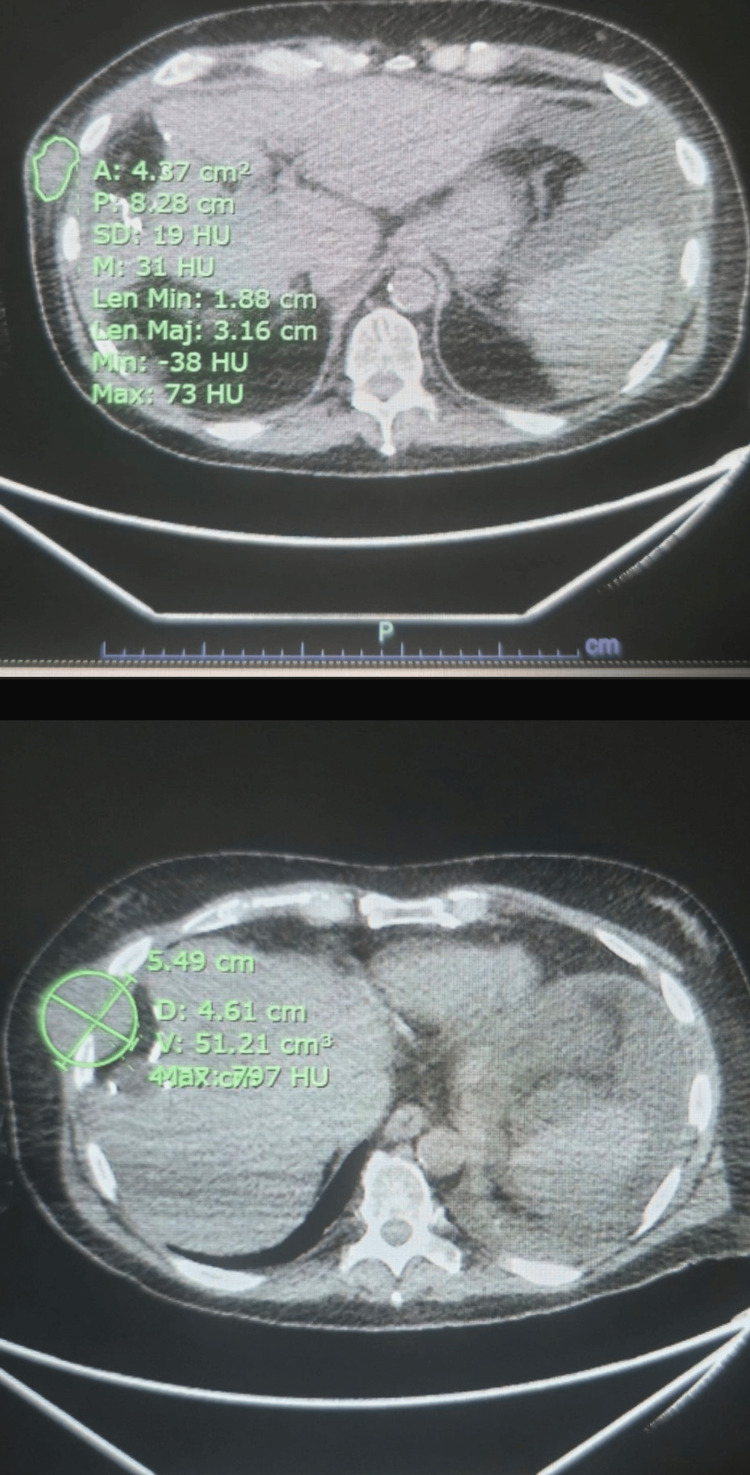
CT images demonstrating chest wall metastasis of HCC with rib involvement and left pleural effusion. CT, computed tomography; HCC, hepatocellular carcinoma
